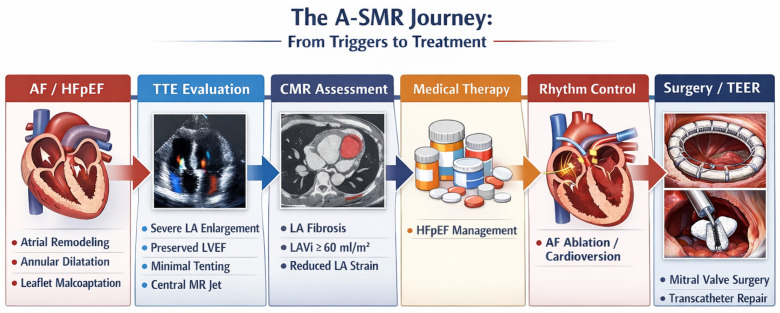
The A-SMR Journey. Abbreviations: AF, Atrial Fibrillation; A-SMR, Atrial Secondary Mitral Regurgitation; CMR, Cardiac Magnetic Resonance; HFpEF, Heart Failure with preserved Ejection Fraction; LA, Left Atrium; LAVi, Left Atrial Volume Index; LVEF, Left Ventricle Ejection Fraction; MR, Mitral Regurgitation; TEER, Transcatheter Edge-to-Edge Repair; TTE, Transthoracic Echocardiography.
